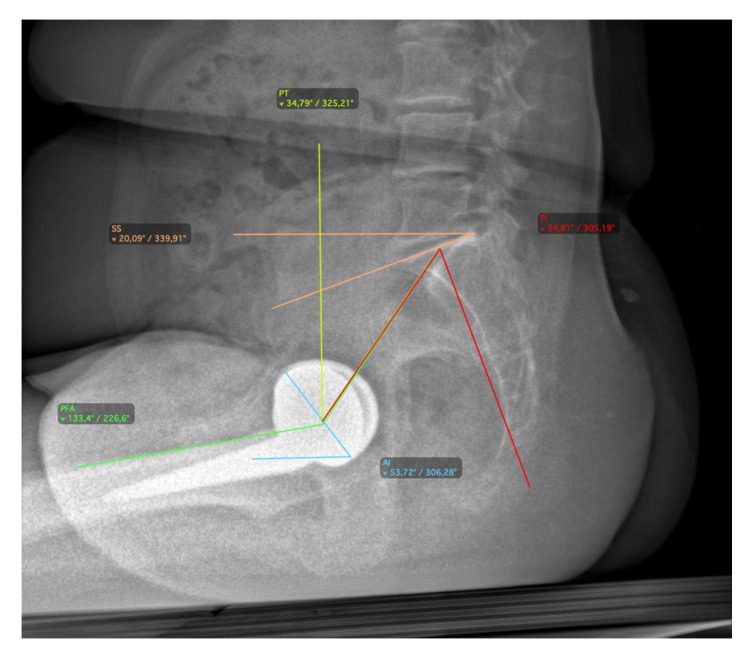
Radiographic measurements for spinopelvic parameters in relaxed-sitting position.
